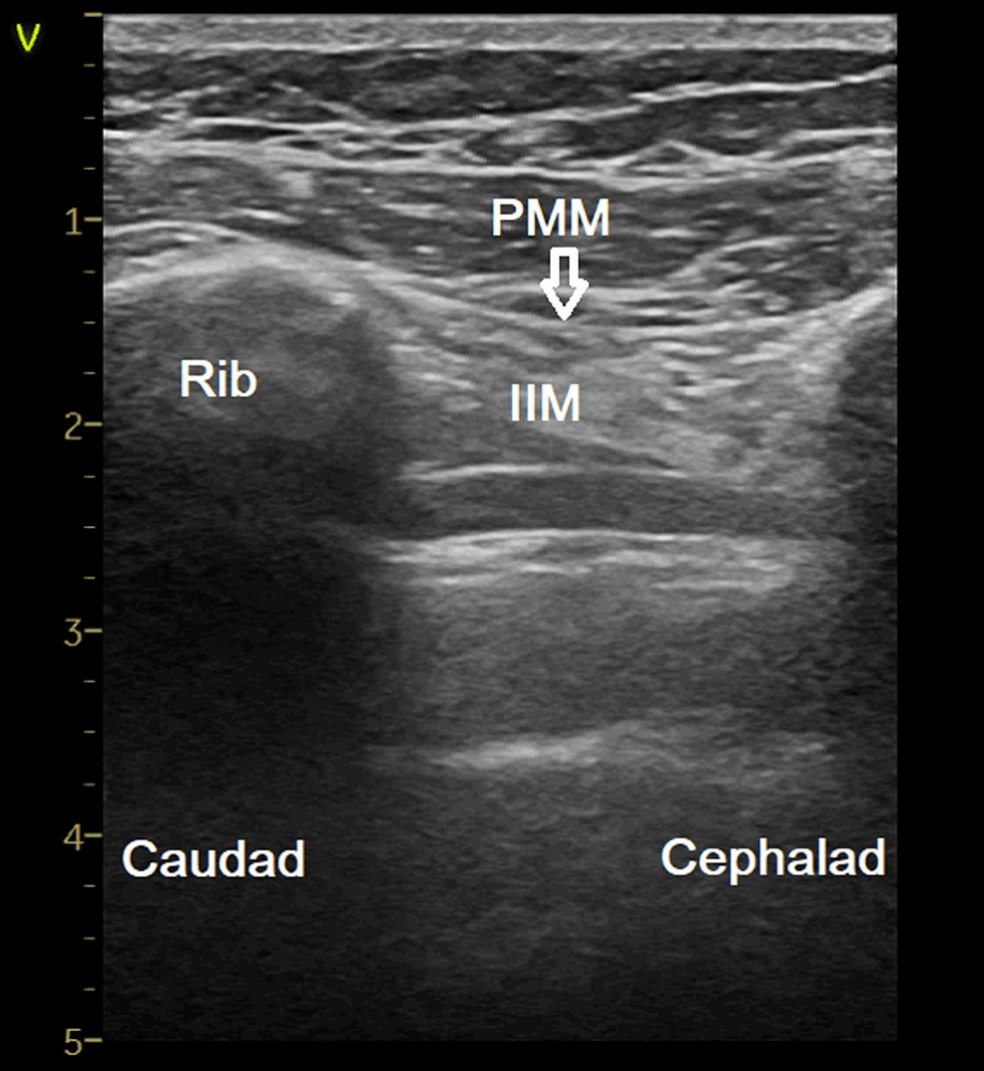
The relevant sonoanatomy for when using an ultrasound-guided superficial parasternal intercostal plane block. The arrow represents the direction of the needle where to inject local anesthetic. PMM: Pectoralis major muscle; IIM: Internal intercostal muscle.
